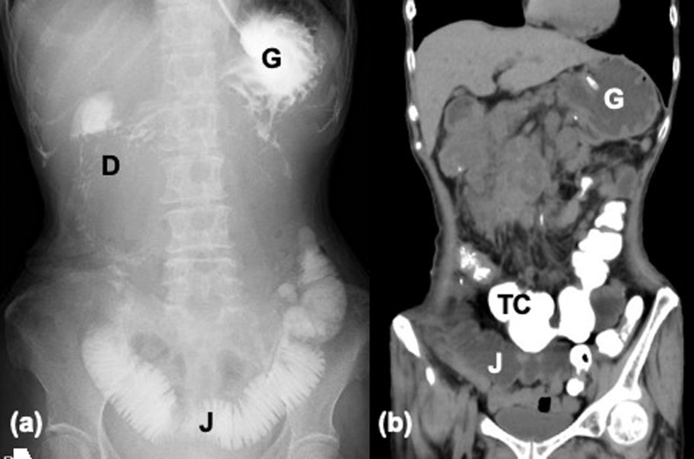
Radiological images after duodenojejunostomy. (a) Gastrografin accumulated in the stomach (G), duodenum (D) and afferent jejunal loop (J). (b) CT scan on the following day showed accumulation of dye in the transverse colon (TC).
